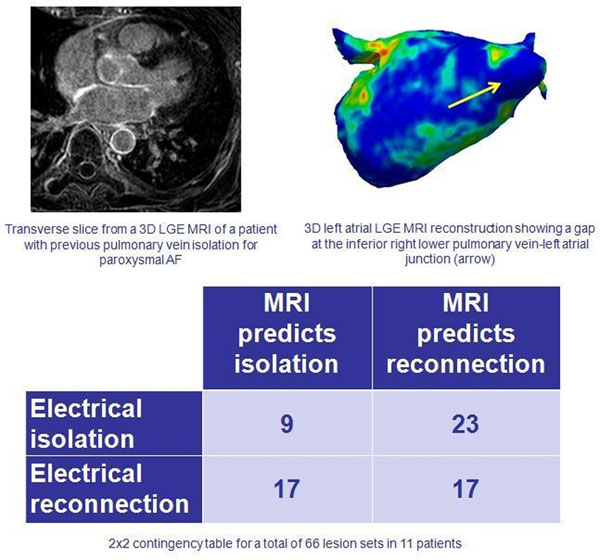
After ablation, three expert blinded reviewers (who were not operators in the repeat procedure) independently scored 3D left atrial (LA) LGE MRI reconstructions (Figure 1) for the presence or absence of gaps at the pulmonary vein-LA junction and across the roof line and mitral line (making a total of 66 lesion sets). Electrical integrity of these lesion sets had been assessed at the time of repeat catheter ablation.
